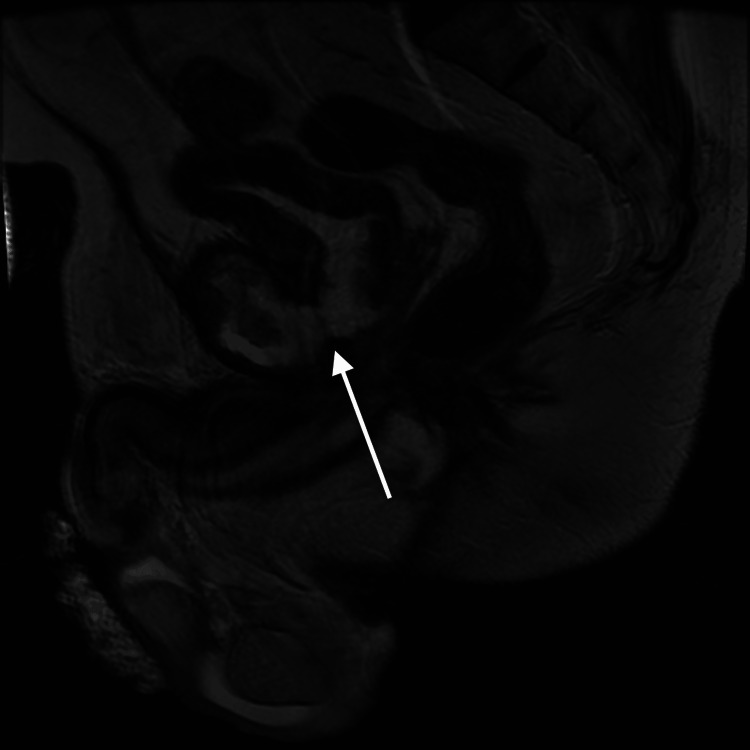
MRI at the initial presentation. The sagittal view shows a fistula tracking from the prostate coursing anteriorly to the symphysis pubis. MRI: magnetic resonance imaging
